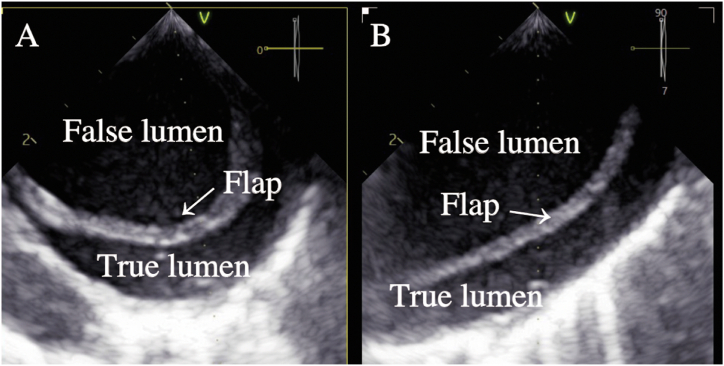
Transesophageal echocardiography images demonstrated dissection of the descending aorta and flap formation at the distal to the left subclavian artery. (A) Short-axis view of the descending aorta. (B) Long-axis view of the descending aorta. The true lumen was compressed by the false lumen.
